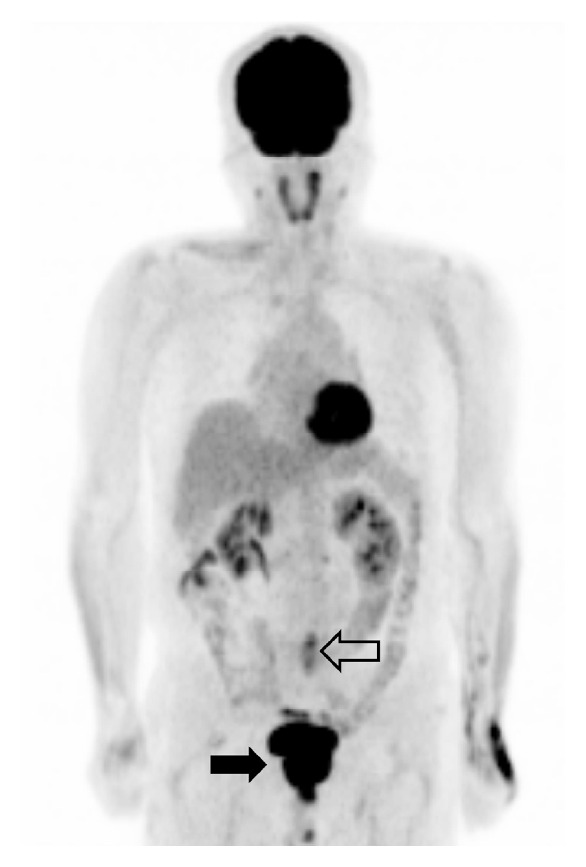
Coronal FDG PET image performed after surgery shows no abnormal uptake suggesting malignancy or metastatic tumor in the body except intense uptake due to prostate cancer and urine in the pelvis (solid arrow) and shows focal increased uptake due to postoperative change in L4/5 level (open arrow).
